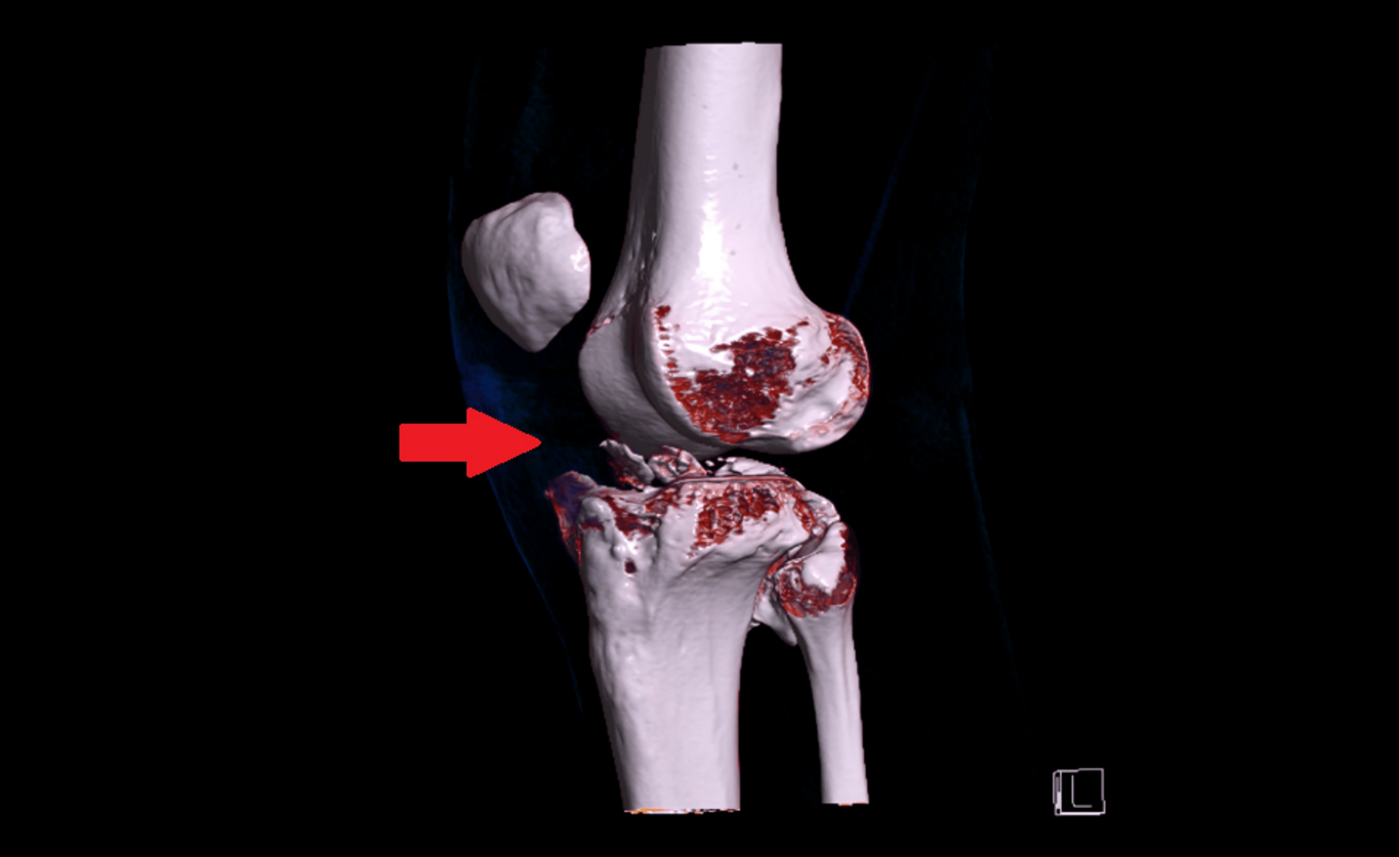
A CT scan showing the fracture with the volume rendering technique (anterolateral view of the left knee)
